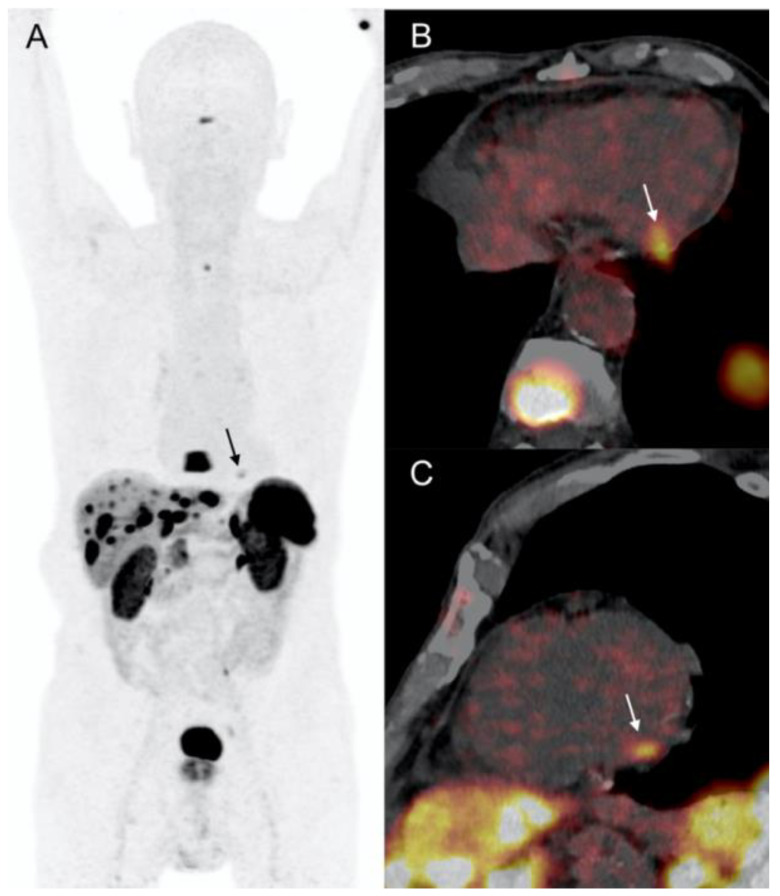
Patient 3. 75-year-old patient with metastatic si-NET and LV CM (arrow). Cardiac lesion showed pathological uptake of ^68^Ga-DOTATOC ((A) MIP anterior projection, (B) PET/CT horizontal long axis, (C) PET/CT short axis).
